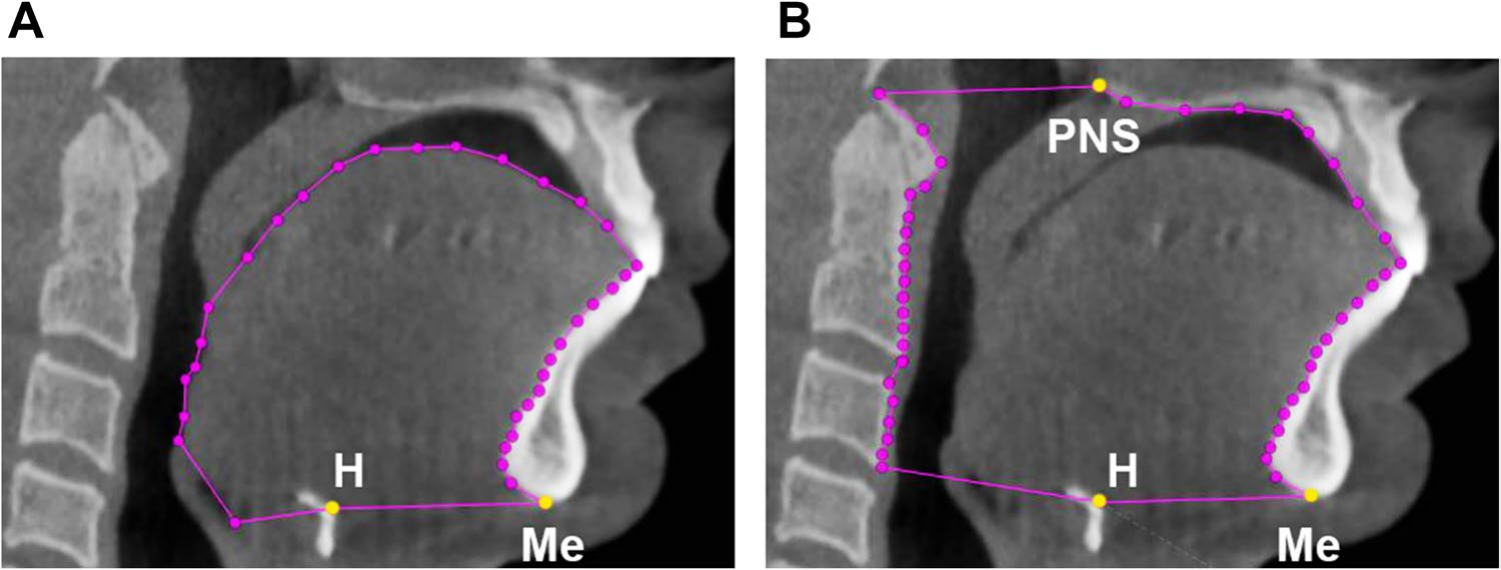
Measurements of tongue size and maxillomandibular enclosure size using cone beam computed tomography (CBCT) imaging in the mid-sagittal plane. (A) Tongue size: area enclosed by the point Hyoid (H), Menton (Me), the contour of the frontal teeth and tongue, and the base of the epiglottis. (B) Maxillomandibular enclosure size: area enclosed by the point Hyoid (H), Menton (Me), the contour of the front teeth, the hard palate, point posterior nasal spine (PNS), and the anterior boundary of the second and third cervical vertebra
